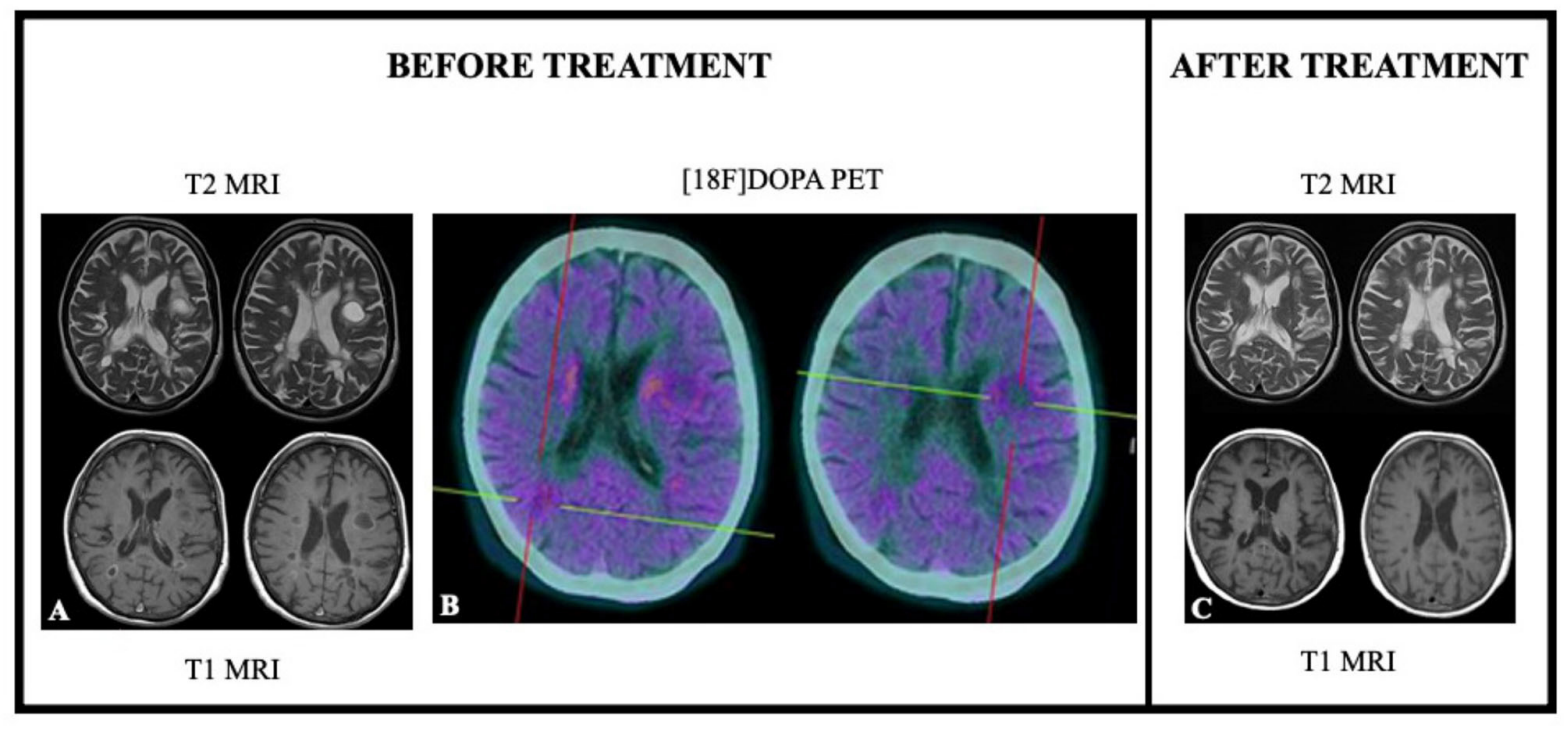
[^18^F]F-DOPA PET and MRI results in a case of differential diagnosis between tumefactive plaque due to multiple sclerosis and brain metastasis from breast cancer. Seventy-one years old female patient previously treated for BC. ceMRI showed the presence of at least two hyperintense at T2 sequences (A) and hypointense at T1 sequences (A) (the largest in left frontal lobe). [^18^F]F-DOPA PET/CT showed a very mild tracer uptake supporting the hypothesis of tumefactive lesion due to multiple sclerosis (B). ceMRI performed 3 months after therapy administration showed reduction in both lesion size and contrast enhancement (C).
